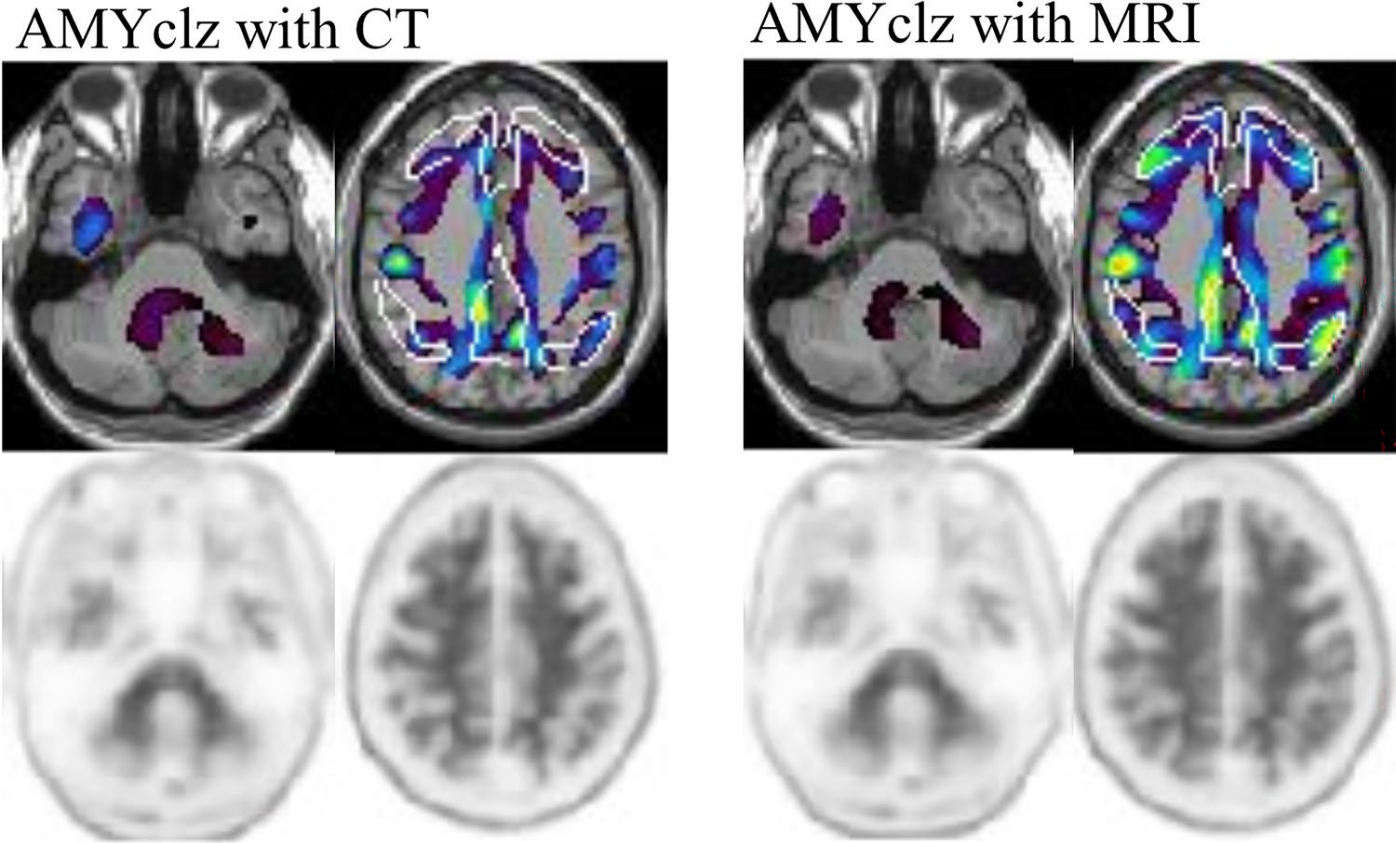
A case where cerebral cortex accumulation appeared to change due to anatomical standardization techniques. The increased accumulation in the precuneus, which was unclear on CT-based imaging, was clearly visible on MRI-based imaging
